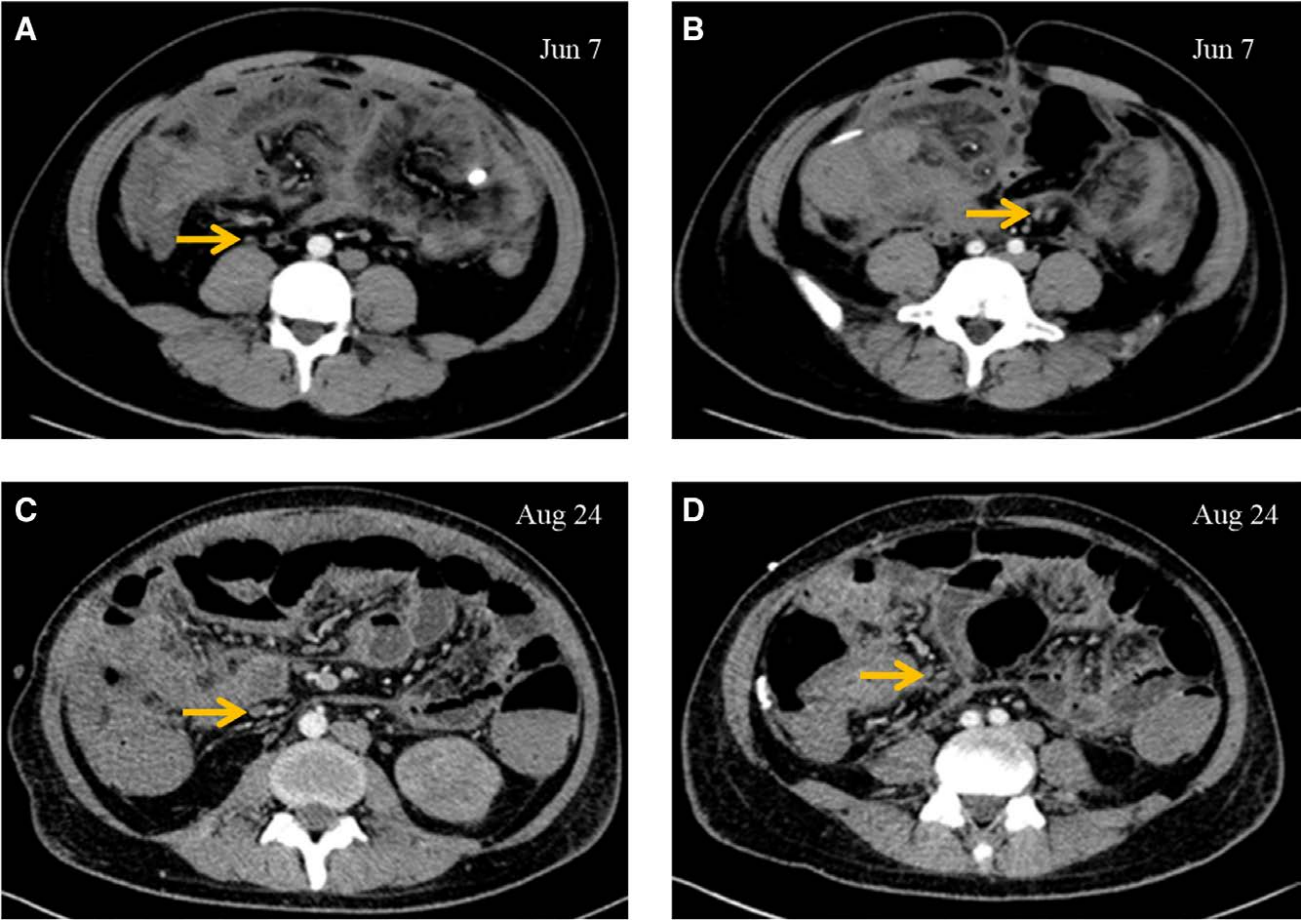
Abdominal computed tomography (CT) scan with contrast abdominal CT scan with contrast on June 7 (A and B) revealed an increase in the size and number of abdominal lymph nodes, which were even larger on August 24 (C and D).
